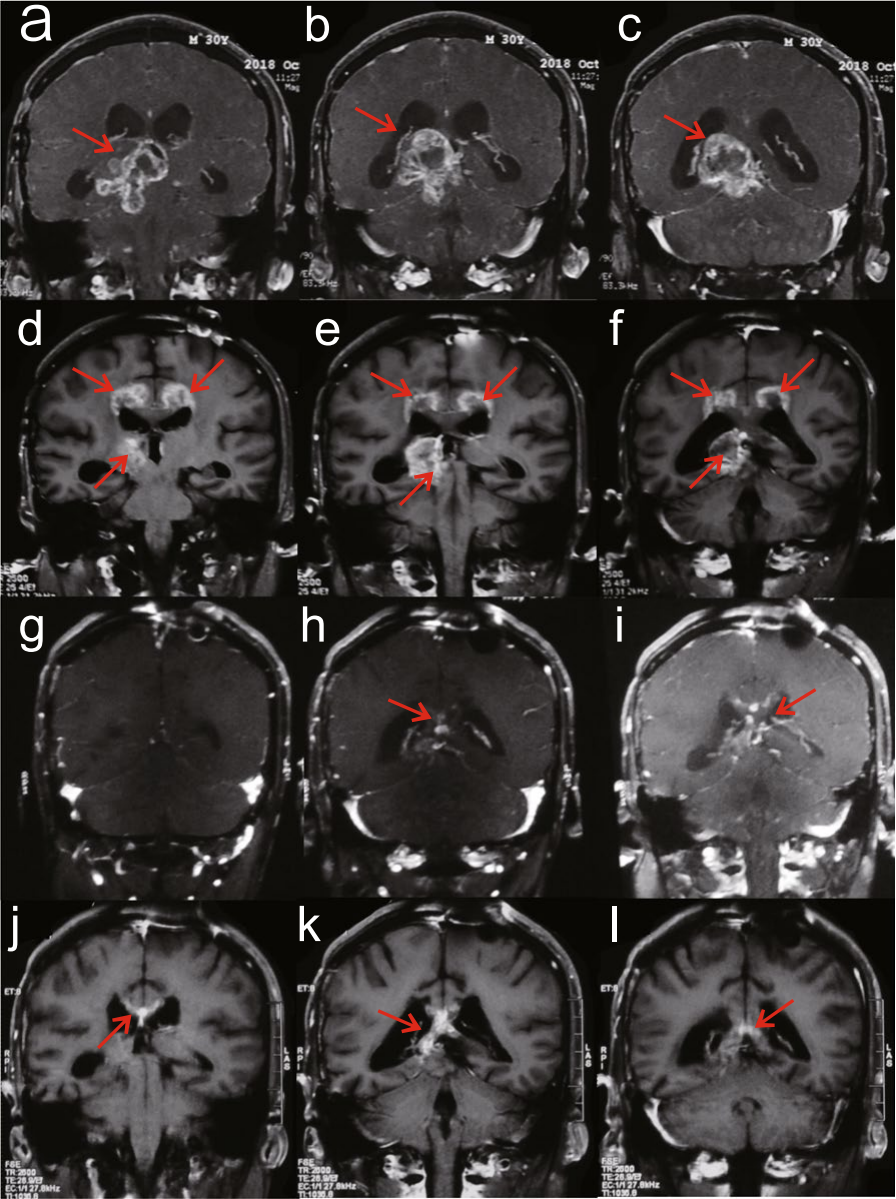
The MRI of one patient who had a partial response. a-c before treatment; d-f after 10 cycles; g-i after 17 cycles, response was PR; j-l when the whole treatment for 2 years was completed (red arrows, tumor)
